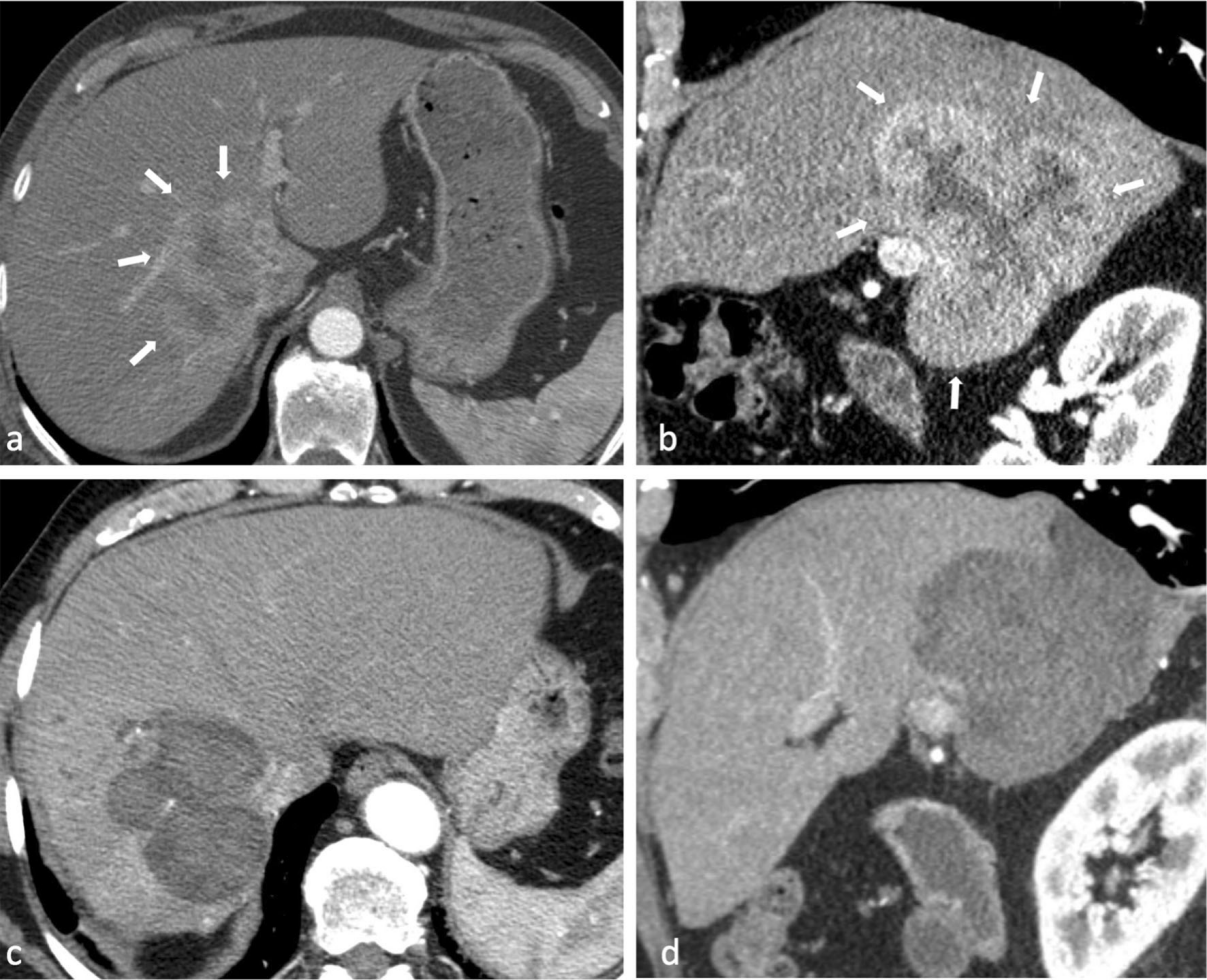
A,B Axial (A) and sagittal (B) plane of contrast-enhanced CT scan showing initial tumor in liver segments I, V, VI, VII, VIII with 10 cm in diameter (white arrows). C, D Axial (C) and sagittal (D) plane of contrast-enhanced CT scan 27 months after the first SRFA (prior to third SRFA session of two distant recurrent nodules) with no residual tumor or local recurrence of initial tumor(s)
